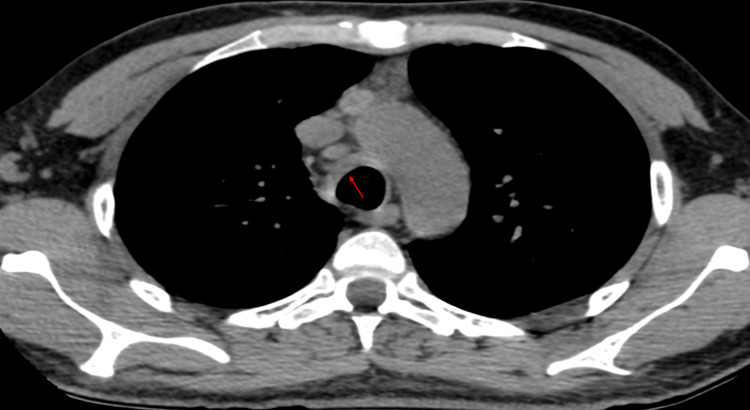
Tracheal tuberculosis CT: computed tomography Axial CT scan showing thickening of the right anterolateral tracheal wall in a patient diagnosed with tuberculosis (red arrow). Diagnosis confirmed by bronchoscopic biopsy of the tracheal wall demonstrating granulomatous inflammation and positive acid-fast bacilli
